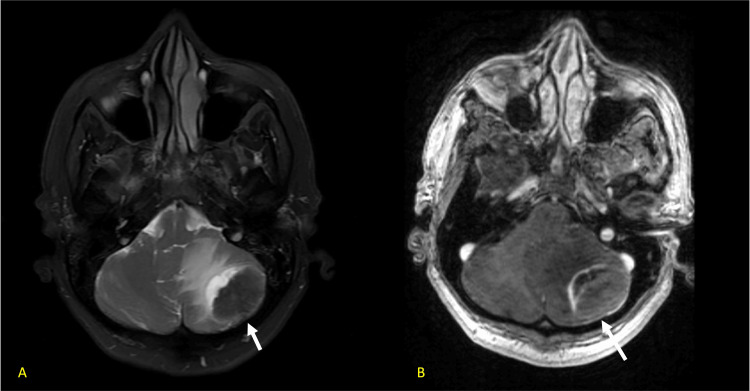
a. T2-weighted axial image through the posterior fossa demonstrating a low signal peripheral mass with adjacent vasogenic edema. b. Post-contrast T1 imaging redemonstrating mass with soft tissue and capsular enhancement.
